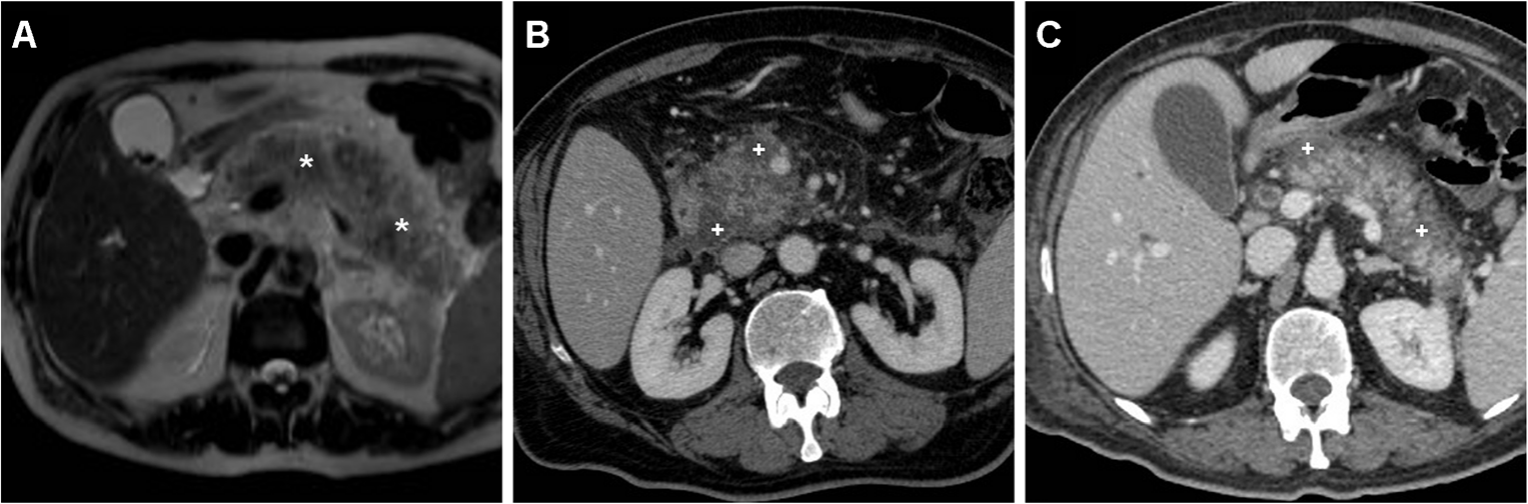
Necrotising AP in a 73-year-old male after ERCP including endoscopic treatment of mild bleeding after papillotomy. Urgent MRI (T2-weighted image in a) requested because of increased C-reactive protein (*CRP*), leukocyte count and serum lipase showed a markedly enlarged pancreatic gland (*) with peripancreatic oedema. Contrast-enhanced CT (b, c) confirmed severe AP with inhomogeneous enhancement of the enlarged pancreas due to diffuse peripancreatic necrosis (+). The patient finally recovered after 2 weeks of in-hospital conservative treatment
